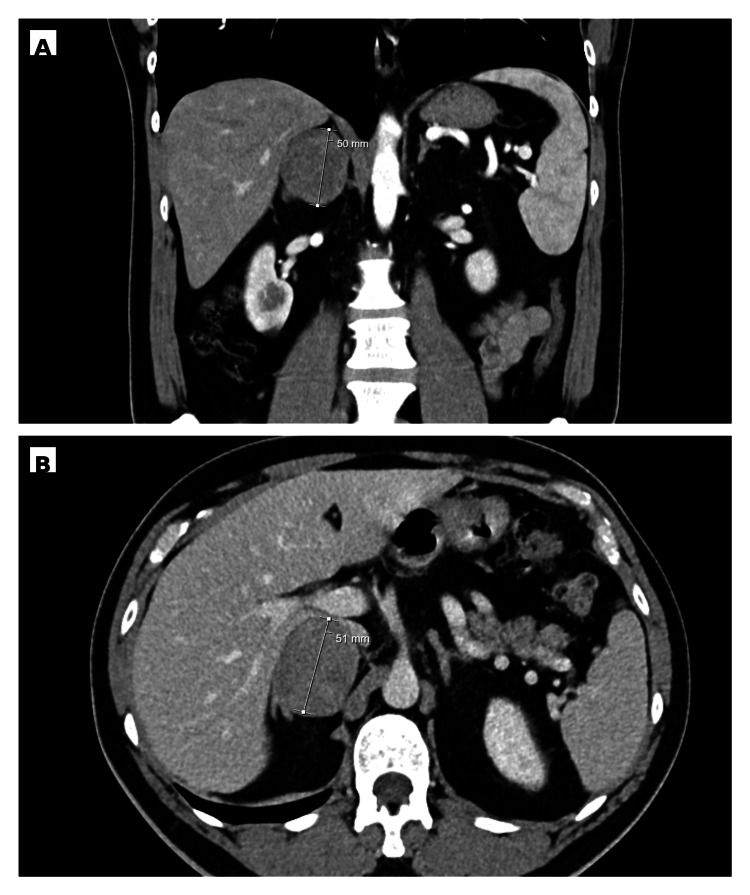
Contrast-enhanced abdominal CT demonstrating a hypervascular right adrenal mass (~5 cm) (A) Coronal reconstruction showing a right adrenal lesion with hypervascular enhancement and mass effect on adjacent structures. (B) Axial image confirming the right adrenal mass, with the maximum diameter measurement displayed
